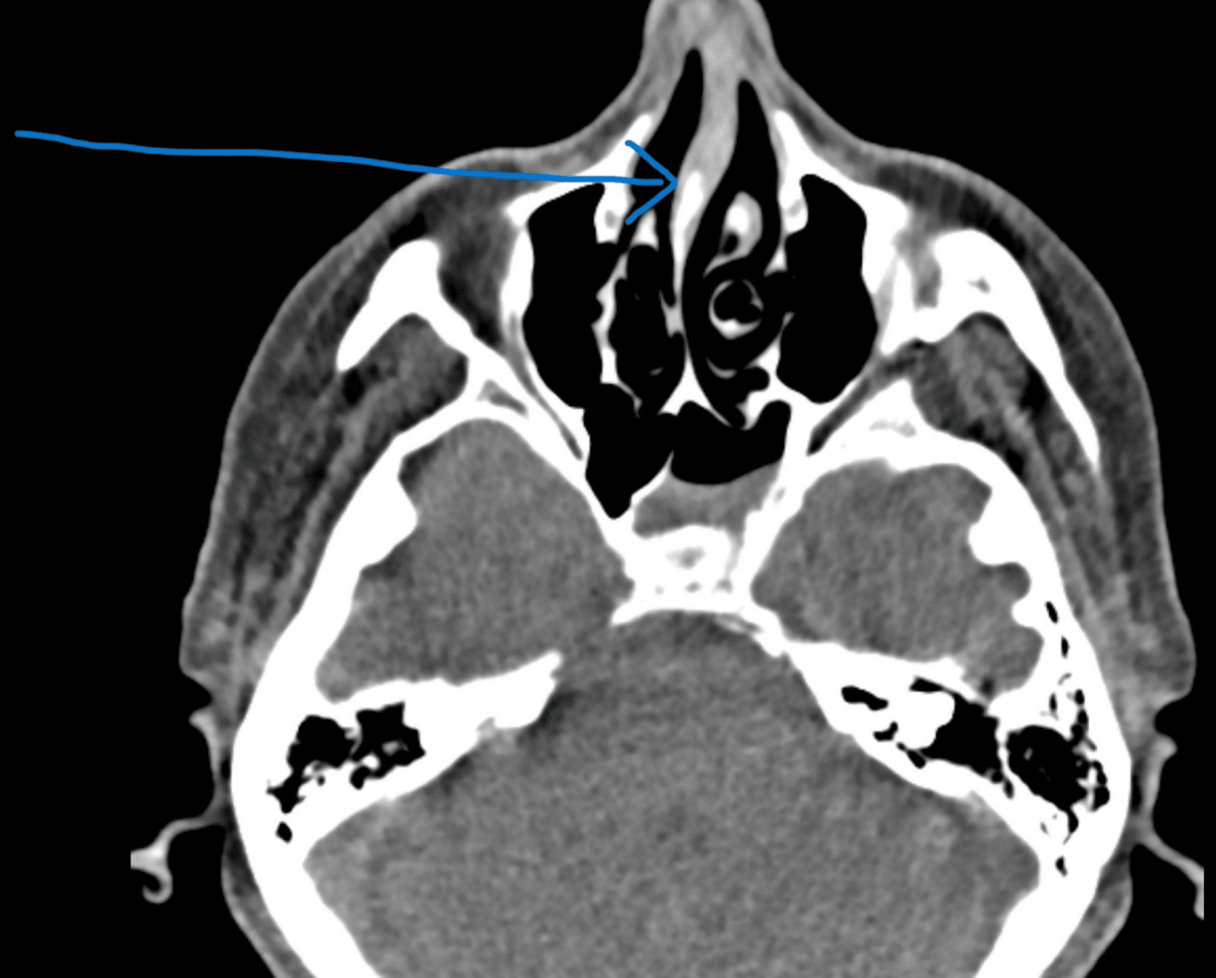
CT scan of the sinuses without contrast showing nasal septum deviation and chronic left sphenoidal sinusitis The arrow shows a deviated nasal septum
